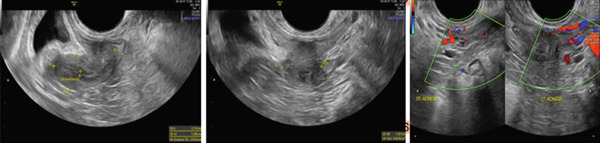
Radiological ultrasound results. Non*cystic* diffuse posterior adenomyosis Type 2–3, heavy, posterior wall thickness 5.53 cm. Uterus anteflexion with homogeneous density, size 4.00 × 2.04 × 1.81 cm, EL 3.63 mm. Bilateral ovaries were difficult to evaluate.
